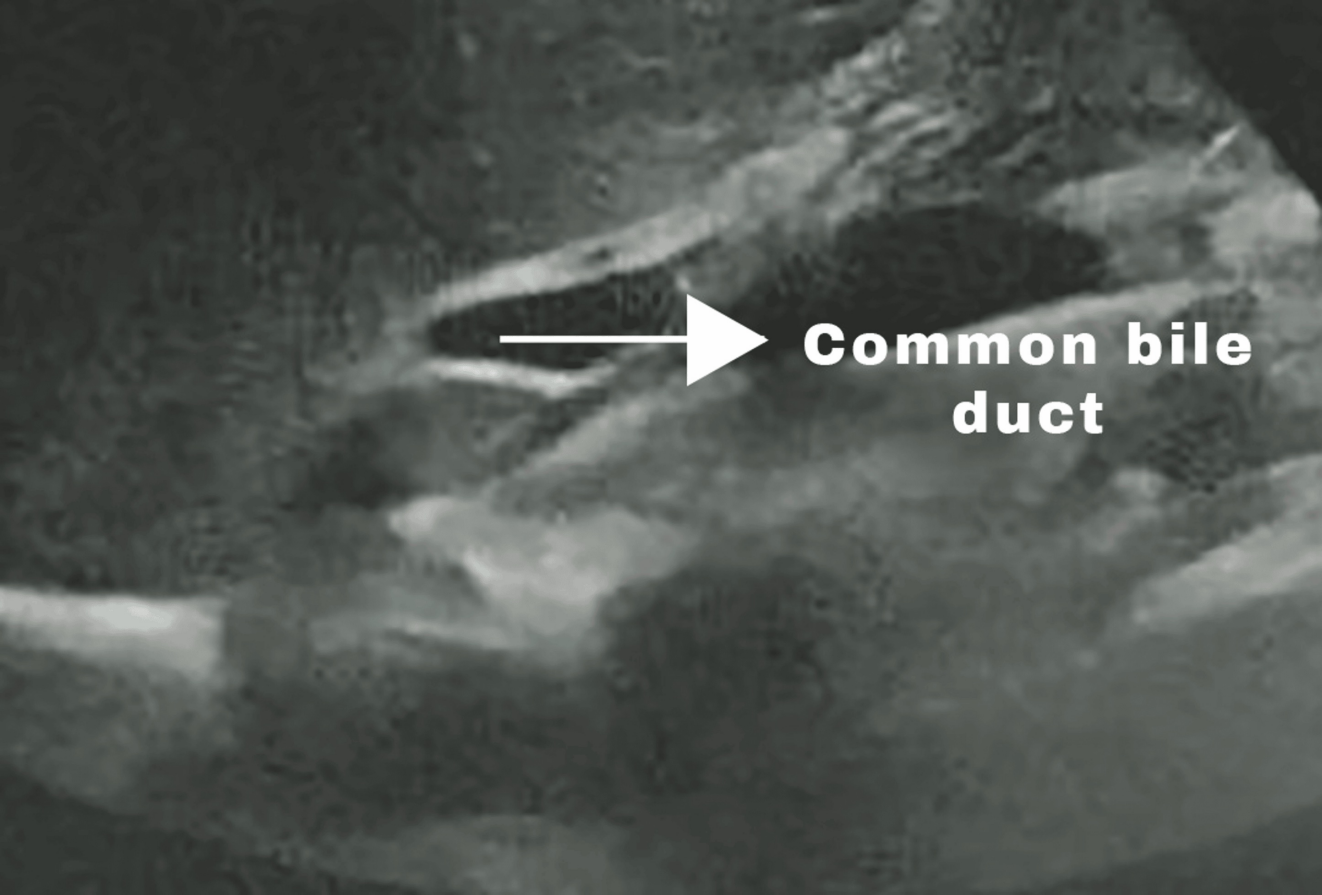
Pre-operative ultrasound abdomen showing normal common bile duct
